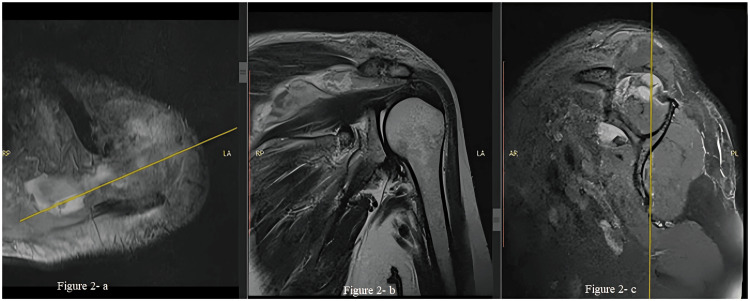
MRI left shoulder showing the fluid collection in the acromioclavicular joint (AC) tracking inferomedially 2-a) Axial cut showing abscess arising from the AC joint. 2-b) Sagittal cut showing destruction of the cartilage of the AC joint with the abscess traveling superior to the supraspinatus muscle. 2-c) Coronal cut showing the abscess arising from the AC joint superior to the supraspinatus muscle with the resultant muscle atrophy; the infraspinatus muscle was not involved.
